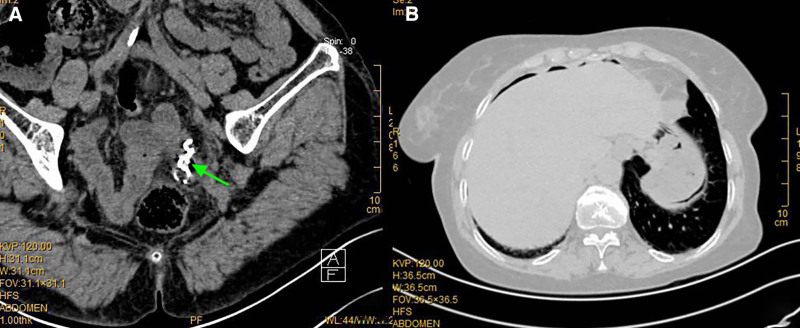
(A) CT showing a high-density foreign body (arrow) in the sigmoid colon. (B) Free air under the diaphragm and gas-distended bowel loops. CT = computed tomography.
